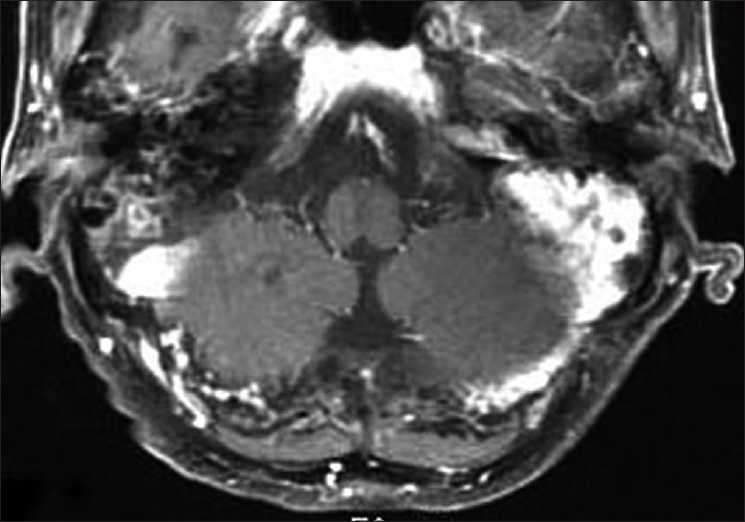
MR image after radiotherapy revealing complete remission
